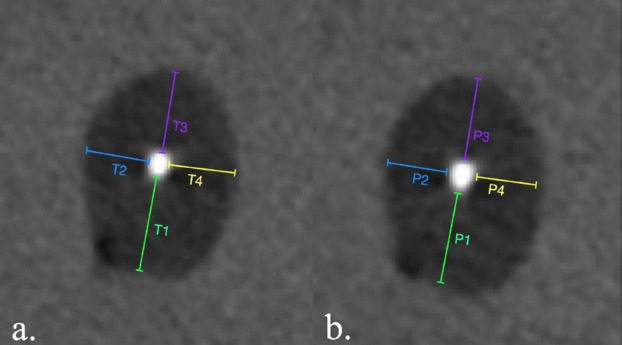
a–b. Axial CBCT slices showing the initial measurements (T1–T4) and the final measurements (P1–P4), obtained before and after instrumentation using the same reference axes.
